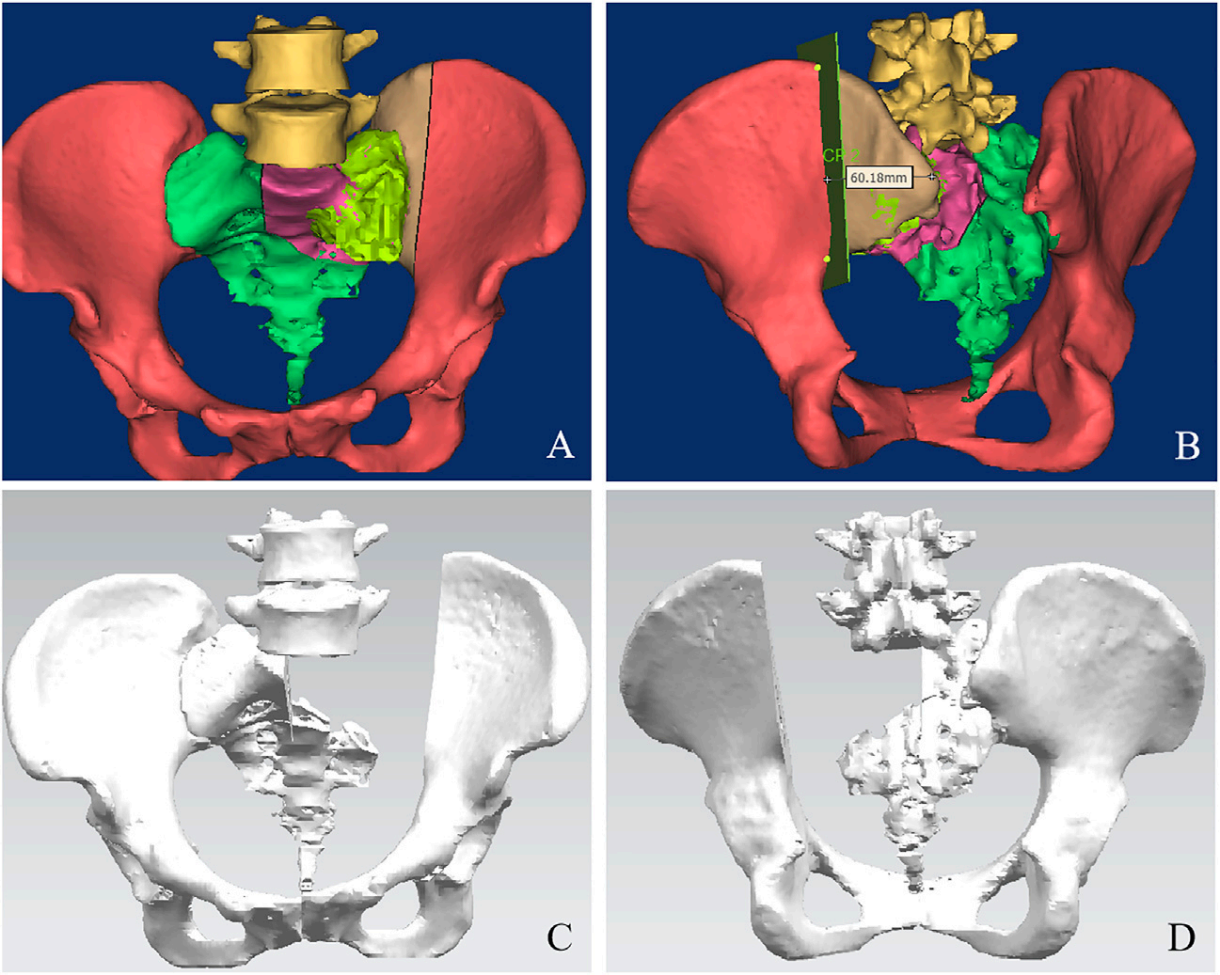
A 3D bone tumor model from CT data was created for surgical planning (A, B). Bone defect model after tumor resection (C, D).
